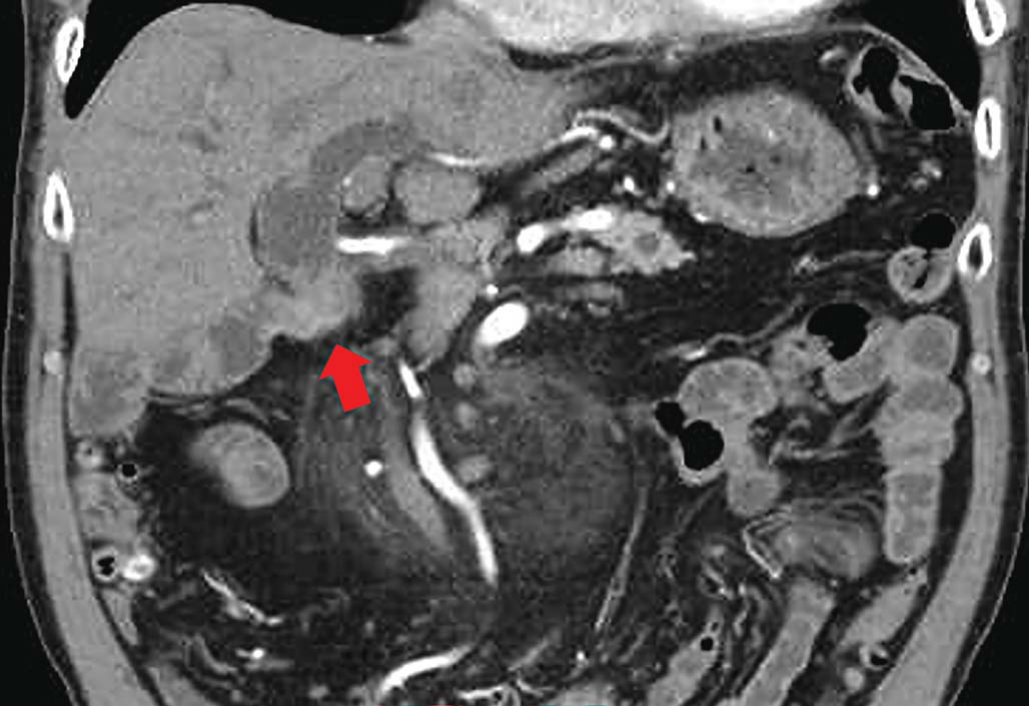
Dilated intrahepatic and remaining common bile ducts and a 23-mm irregular intra-abdominal mass at the choledochojejunal anastomotic site (CAS) were detected on computed tomography (CT). A red arrow shows a 23-mm irregular intra-abdominal mass at the CAS.
